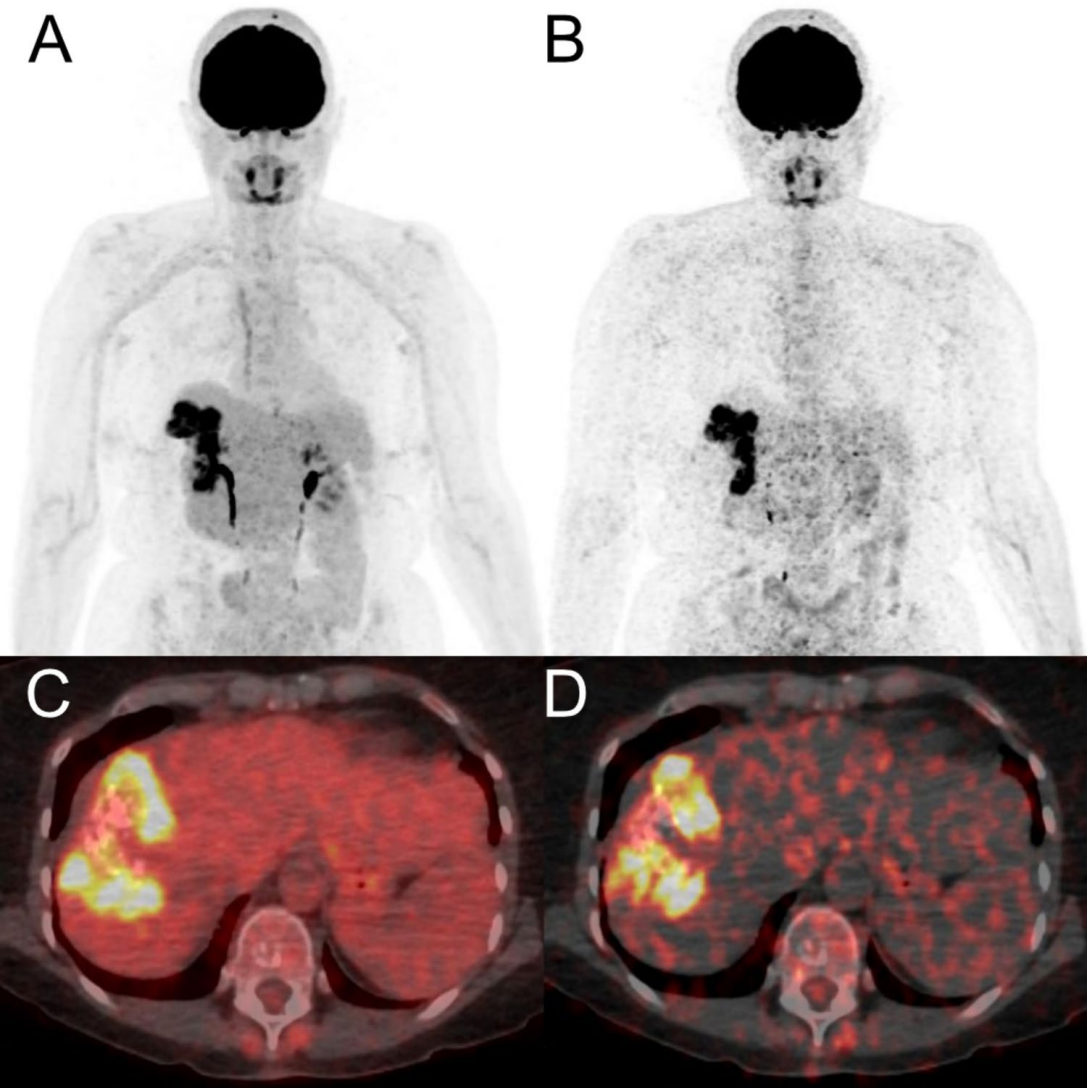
PET/CT performed for therapy monitoring of AE in a 55-year old woman (patient 06 in Table 1) showed intense metabolic activity (in maximum intensity reconstructions of PET, A and B) and fused PET/CT images (C and D) in a partially calcified lesion in the right liver lobe. In standard static PET/CT images (A and C), SUVmax of the AE manifestation was 11.2, and the lesion-to-background ratio (SUVratio) was 2.7. For WBD PET/CT (B and D), the respective value in the AE manifestation was 43.3, and the lesion-to-background ratio was comparatively higher (Patlak ratio 4.3)
